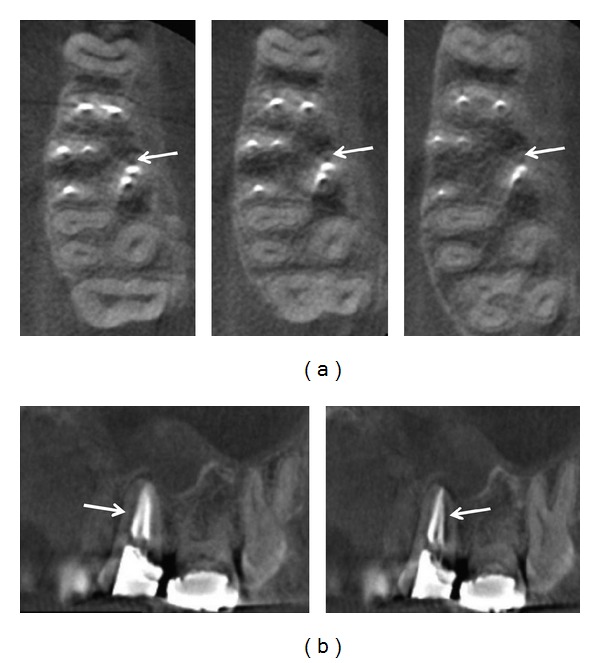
(a) From left to right, axial scans of coronal, middle, and apical root sections suggesting the presence of a third root canal in the palatal root (arrows). (b) Sagittal scans demonstrating that the third canal observed at previous images was an artifact (arrows). Note the presence of hypodense periapical areas on the palatal root.
